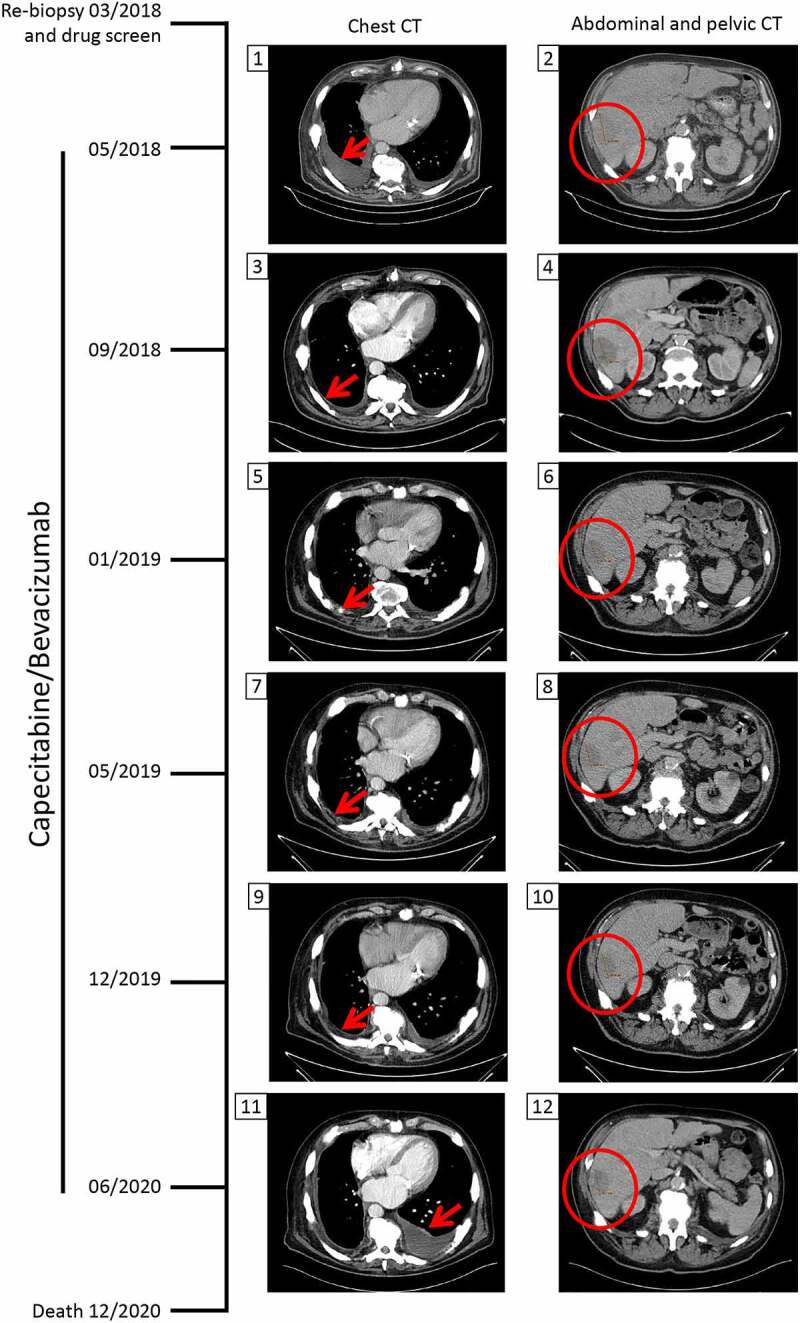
Chest and abdominal/pelvic computed tomography (CT) scans during capecitabine/bevacizumab treatment. After evidence of progressive disease, the patient underwent a re-biopsy in March 2018 for *ex-vivo* drug screening. In May 2018, CT scans revealed pleural effusion due to pleural carcinosis and progression of liver metastases. The tumor board decided to treat the patient with capecitabine and bevacizumab based on the personalized functional profiling results and on reported efficacy of this combination in NET. In September 2018, the pleural effusion/pleura carcinosis regressed and a stable disease regarding liver metastasis was noted. With this treatment regimen, the patient maintained a stable disease until its progression in June 2020. The patient died 6 months later. The red arrow shows the appearance, regression and re-appearance of pleural effusion. The red circle marks the liver tumor mass.
